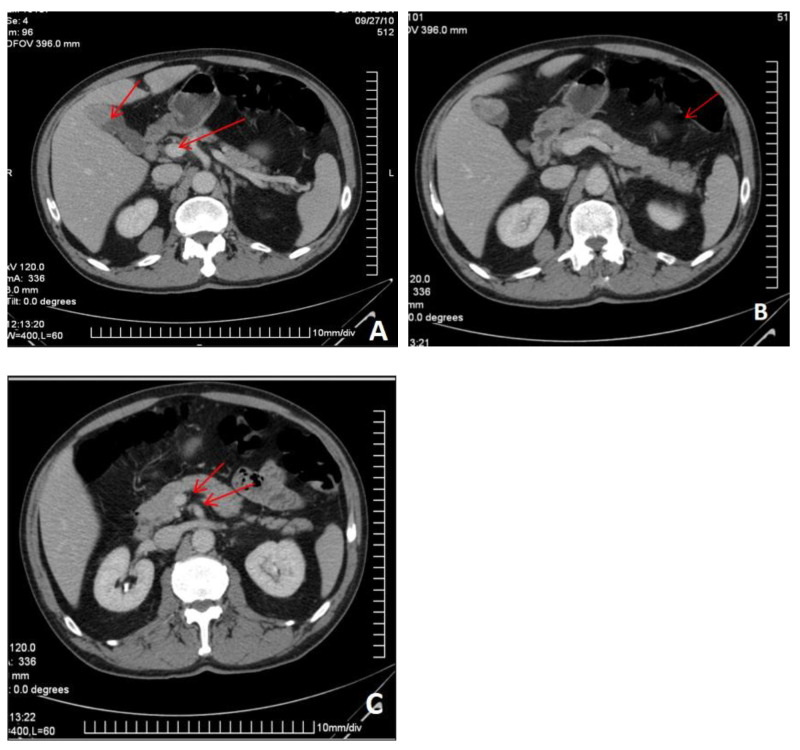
Axial enhanced abdominal portal-venous phase CT images showing a gallbladder mass (A), a pancreatic type enhancing mass (B), and retroperitoneal lesions (C), all highly suggestive of metastasis (arrows).
